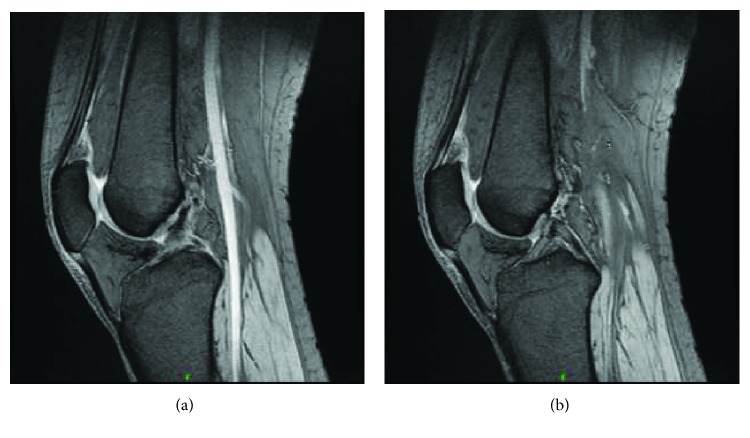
(a) Lateral-view T2 sequence MRI of the left knee 6-month post arthroscopic resection of posterior-compartment PVNS, showing no more compressive effect on both the cruciate ligaments and the popliteal vessels. (b) There is a significant decrease in the soft tissue enhancement suggestive of decreased inflammation in the region of the resected lesion. There is no evidence of interval appearance of new susceptibility artifacts or blooming effect suggesting recurrence.
